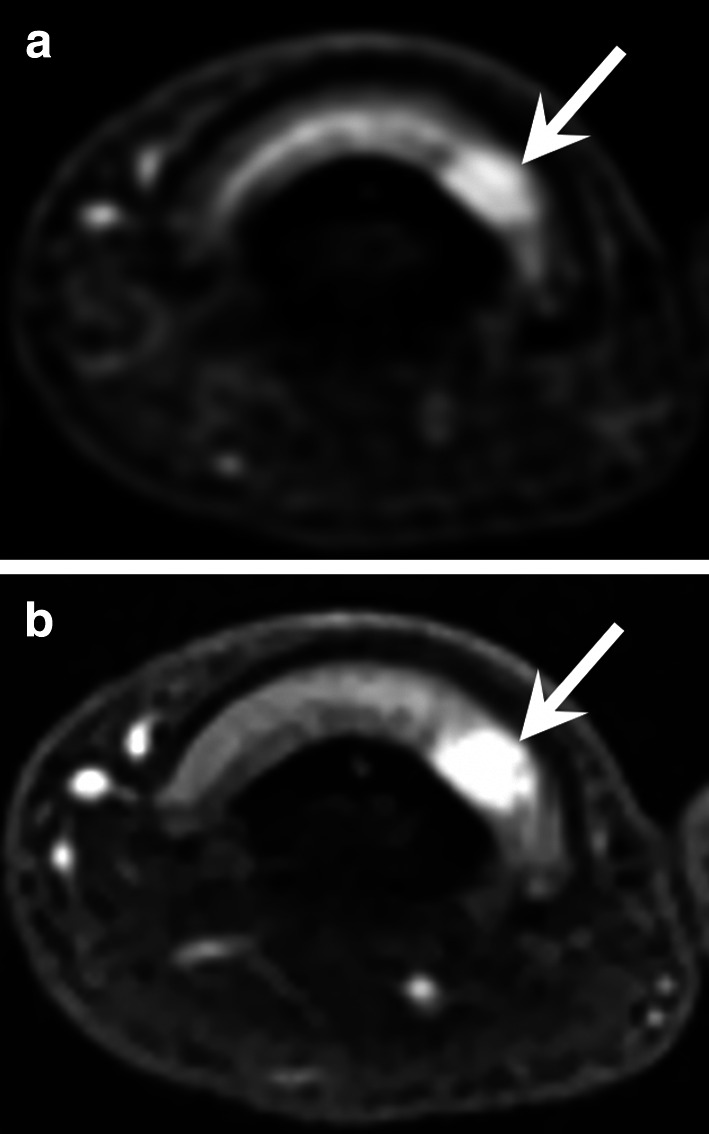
Glomus tumour of the third finger in a 32-year-old woman. a, b Axial T2-weighted MR images and contrast-enhanced T1-weighted fat-suppressed sequence showing a small hypervascularised nodule (arrow) of the ulnar side of the nail matrix
